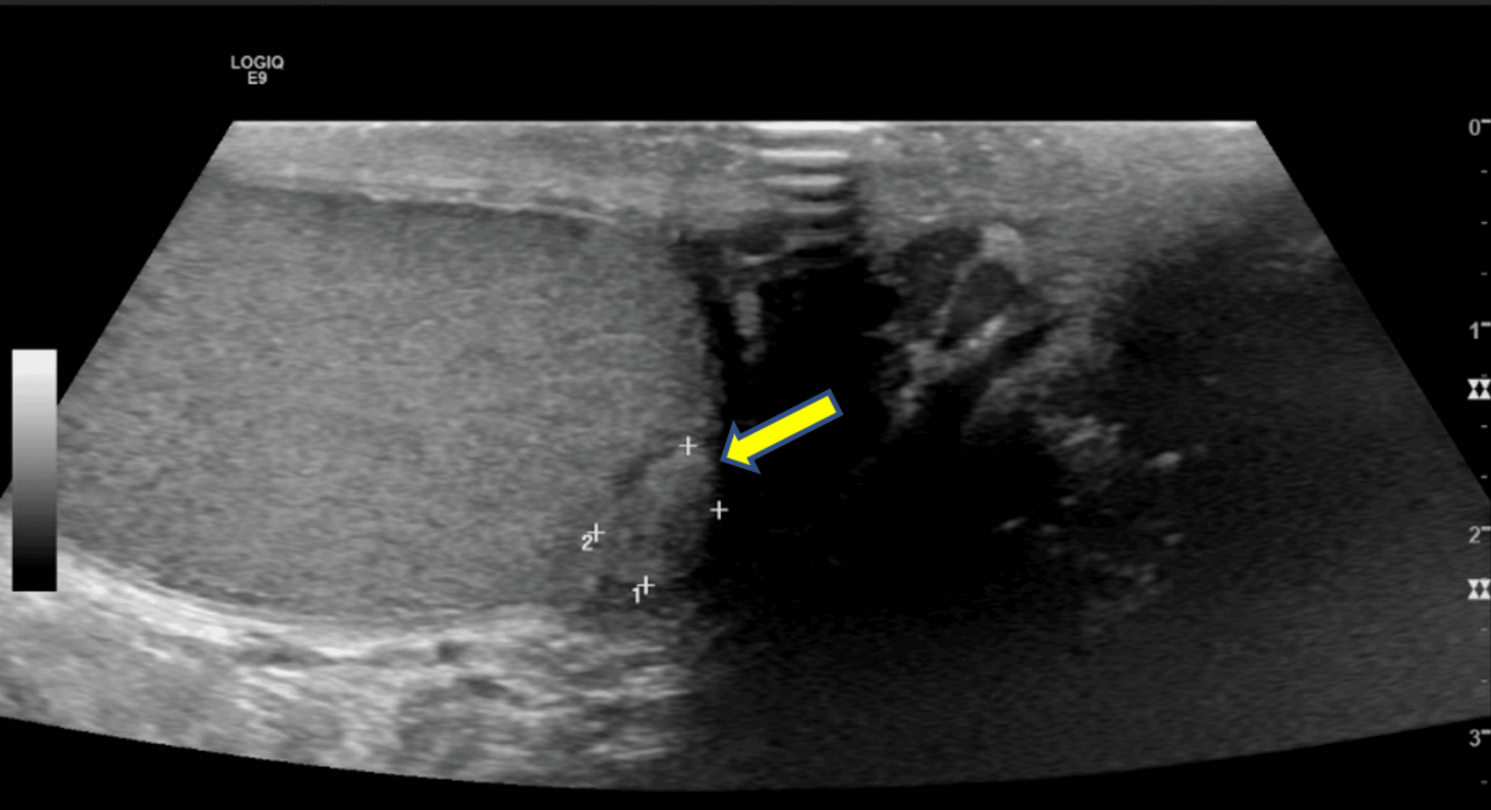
Scrotal ultrasound showing the left intratesticular peripheral hypoechoic lesion measuring 0.7 x 0.6 cm with no internal vascularity (yellow arrow).
